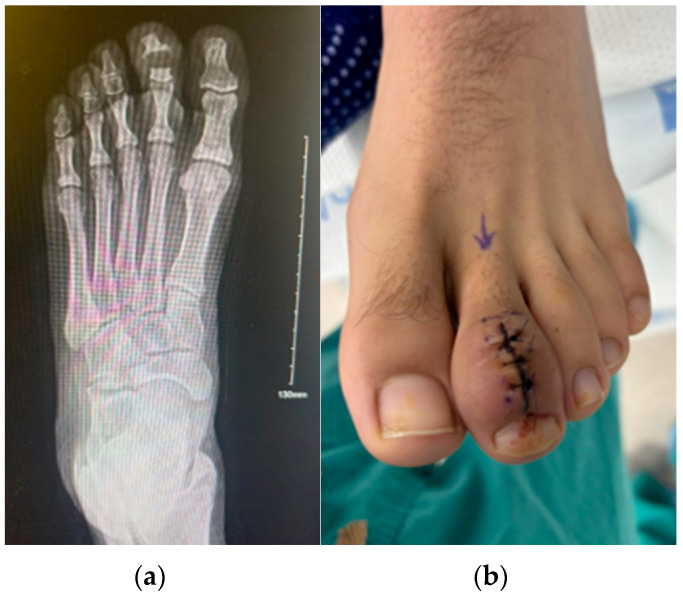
(a) Post-bone biopsy radiographs. (b) Clinical photograph demonstrating persistent swelling and structural changes.
